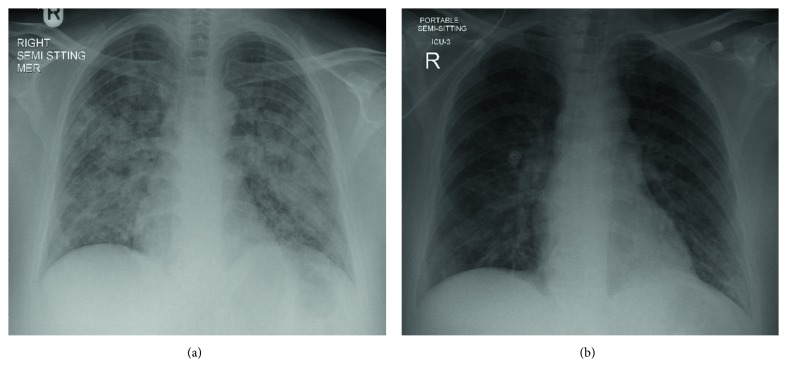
(a) CXR on admission showing multiple confluent and patchy air space opacities noticed diffusely involving both lung fields. (b) CXR after 2 days showing the bilateral lung opacities appear significantly resolved as compared to the previous chest radiograph.
